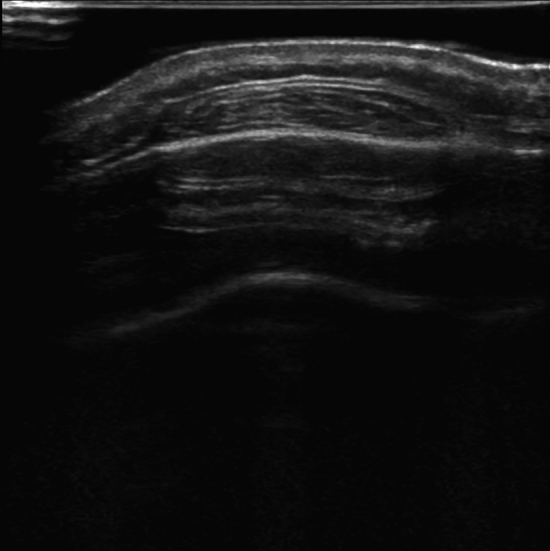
Ultrasound results of forehead lipoma
